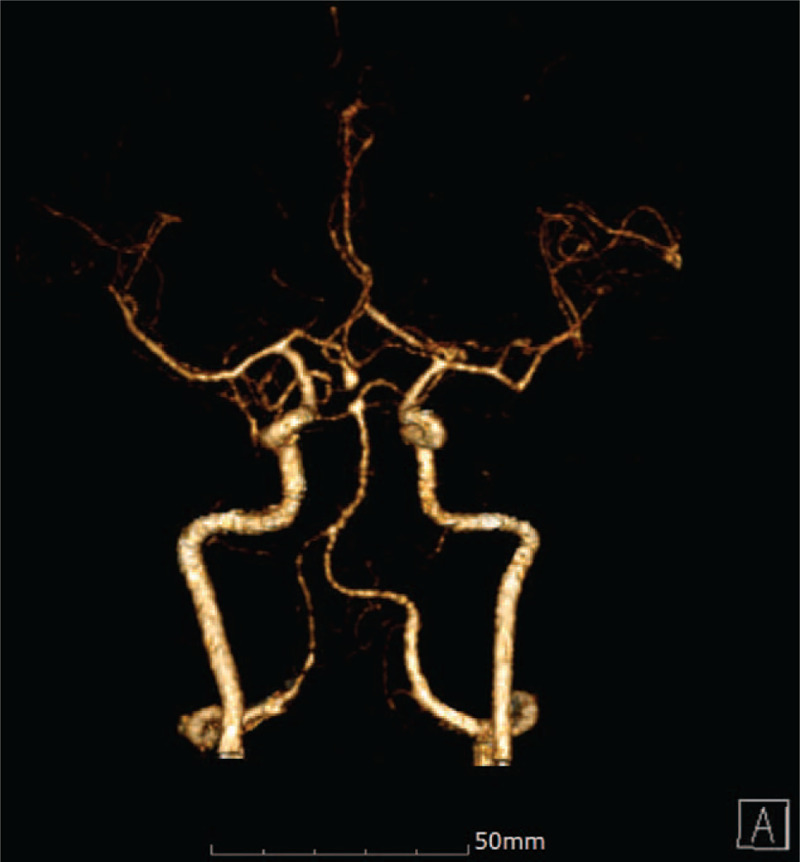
Three-dimensional brain CT angiography showed a right feeding anterior communicating artery aneurysm. CT = computed tomography.
